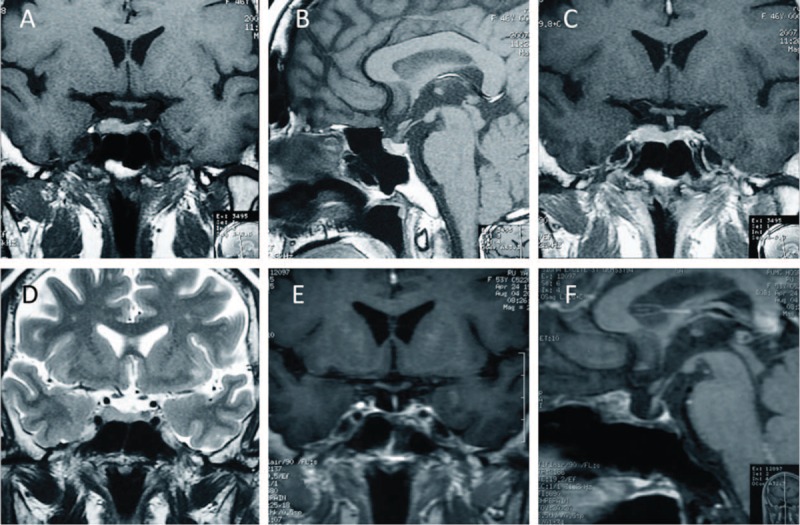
Magnetic resonance imaging (MRI) shows an abnormal enlarged parenchymal lesion in the sellar region.
